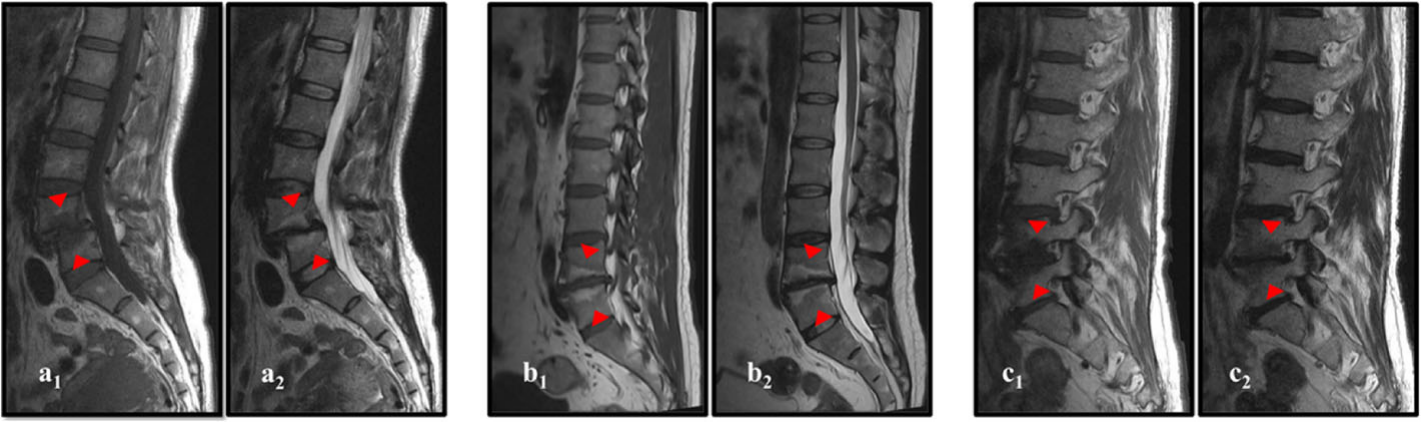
Representative T1- and corresponding T2-weighted images of different MCs. The red arrows indicate the positions of the MCs. MCs, Modic changes
